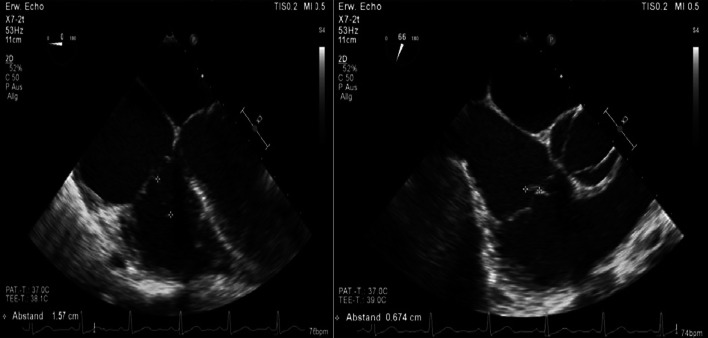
Transoesophageal echocardiography showing a decrease in vegetation on the septal leaflet of the tricuspid valve
